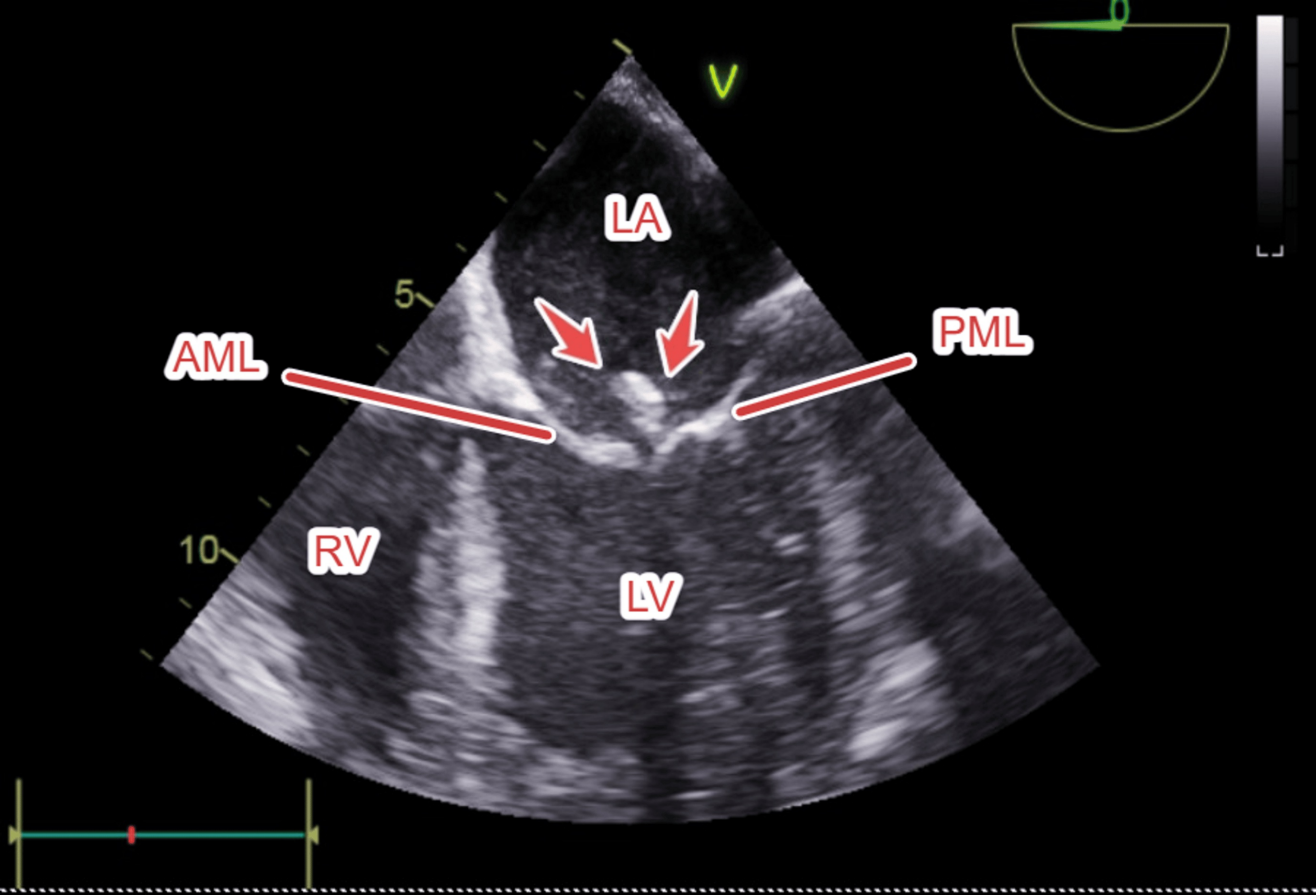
Mid-esophageal four-chamber TEE view at 0°, showing a mobile echogenic mass (red arrow) attached to the PML, consistent with mitral valve vegetation LA, left atrium; LV, left ventricle; RV, right ventricle; AML, anterior mitral leaflet; PML, posterior mitral leaflet; TEE, transesophageal echocardiography
